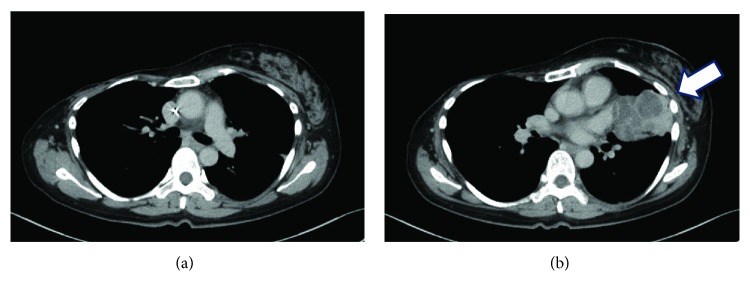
Computed tomography scan acquired after docetaxel chemotherapy showing (a) a lack of reappearance of the right lung mass and (b) an increase in the size of the left lung mass to 5.5 cm (arrow).
